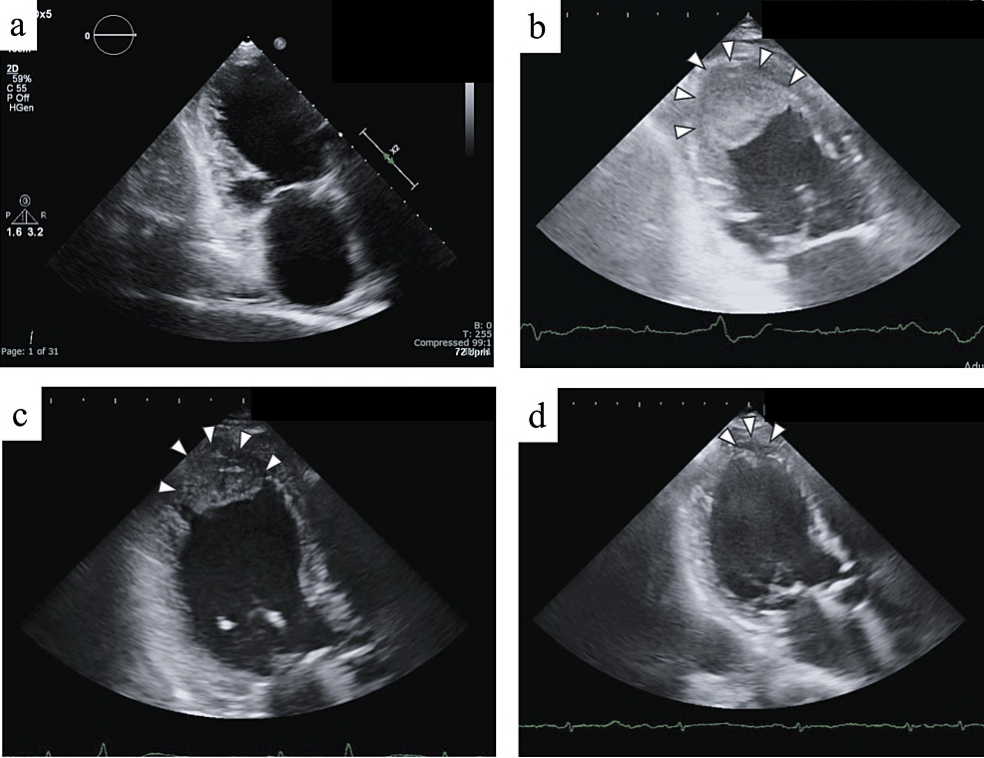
Time course of thrombus images by echocardiography Echocardiographic images approaching from the apex of the heart. This is characterized by a regular shape, uniform echogenicity, and no evidence of mobility, consistent with a fresh thrombus image. (a) Echocardiographic image obtained 40 days before admission. An enlarged left ventricle is seen with a spherical apex without a thrombus. (b) Echocardiographic image obtained seven days after admission (immediately after the onset of the disease). A large thrombus of size 30×18 mm (white arrowheads) is seen in the left ventricular apex. (c) Echocardiographic image obtained on the 14th day after admission (seven days after the onset of the disease). The thrombus appears to have shrunk to 27×13 mm (white arrowheads) with anticoagulation therapy. (d) Echocardiographic image obtained on day 42 after admission (day 35 after the onset of the disease). The thrombus appears to have shrunk to 12×8 mm (white arrowheads).
